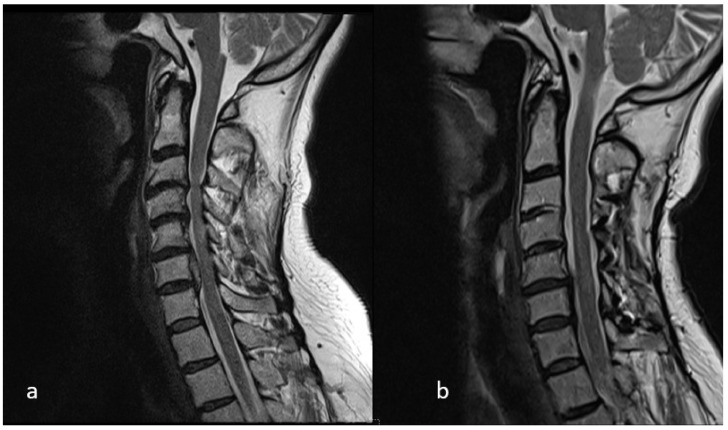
The levels selected for laminoplasty were determined preoperatively based on the extent of spinal cord compression identified on MRI: (a) based on the preoperative MRI, it was decided to perform laminoplasty at the C3–C4–C5–C6 levels; (b) postoperative MRI showing that the canal had relieved.
